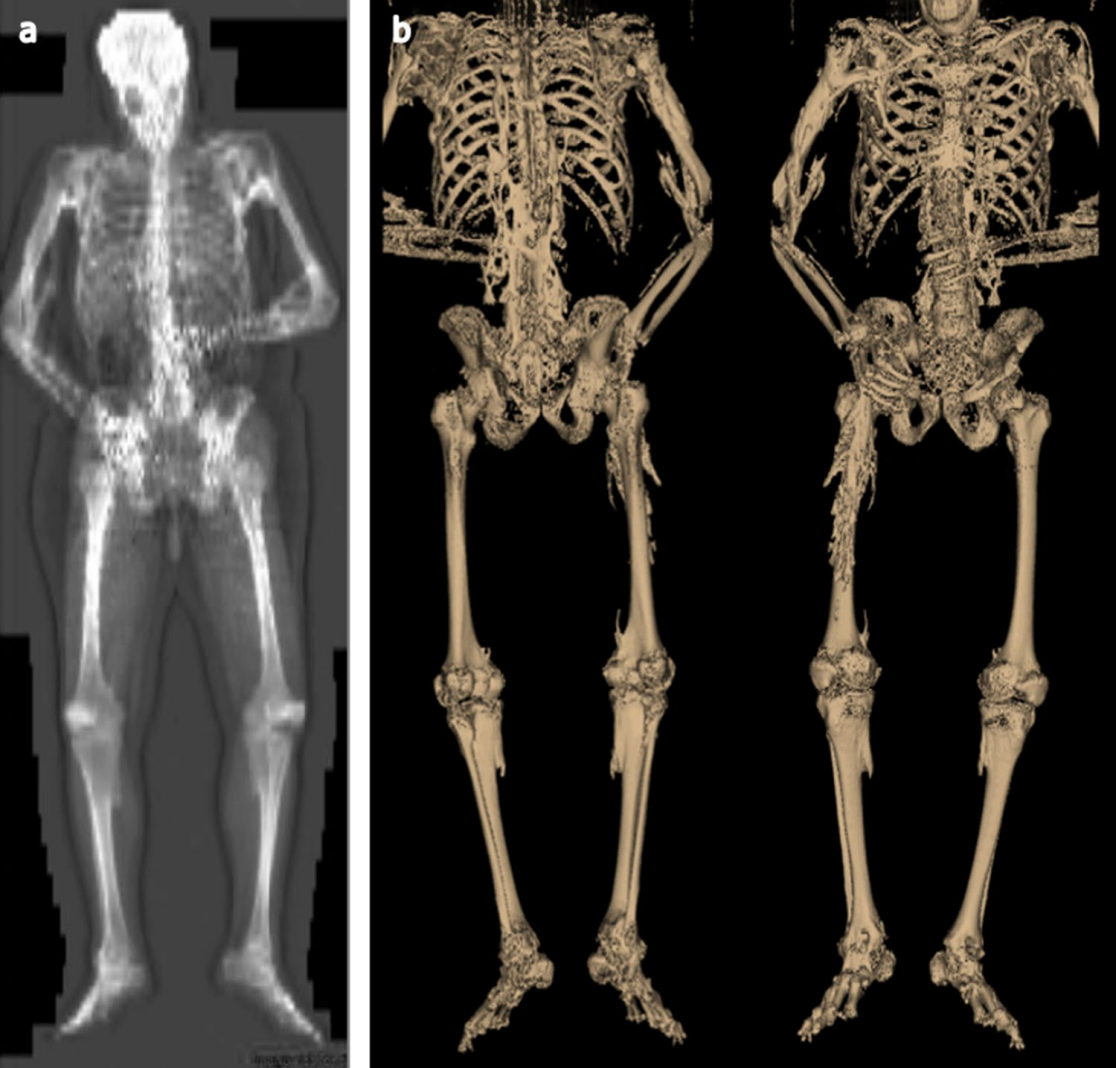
Representative a DXA and b 3D reconstructed WBCT scan (for demonstration only). Images were taken from the same 33-year-old male, with a Baseline CAJIS score of 18 at enrollment. The 3D reconstructed WBCT scan images were not used in the assessment or quantification of new HO in the analyses presented here, but are provided to demonstrate the amount of HO in a patient with FOP and to provide a similar view to the DXA images. *3D* three-dimensional, *CAJIS* Cumulative Analogue Joint Score, *DXA* dual energy X-ray absorptiometry, *FOP* fibrodysplasia ossificans progressiva, *WBCT* whole-body computed tomography
